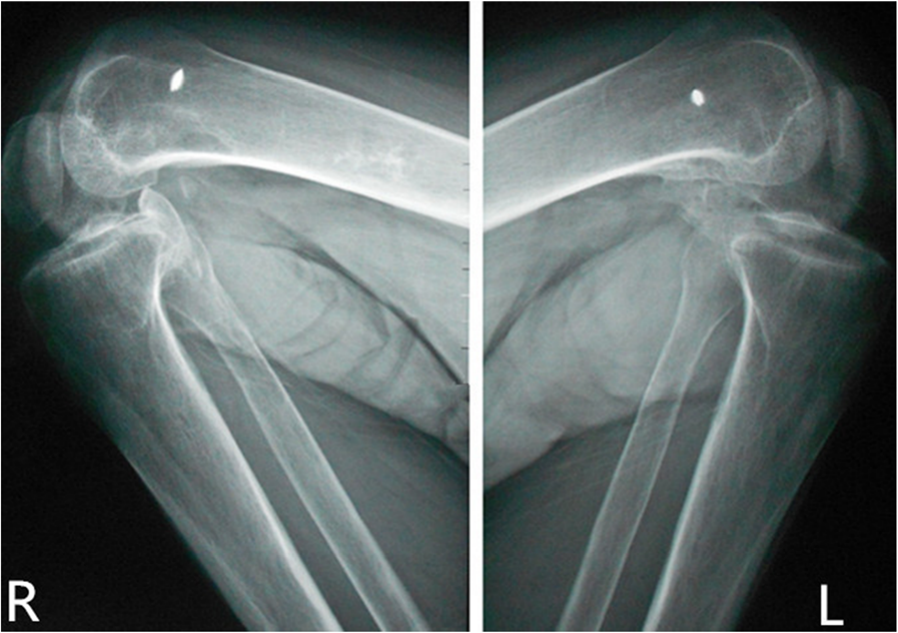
Postoperative radiographs of knees shows the passive range of motion of knees had significantly improved.
